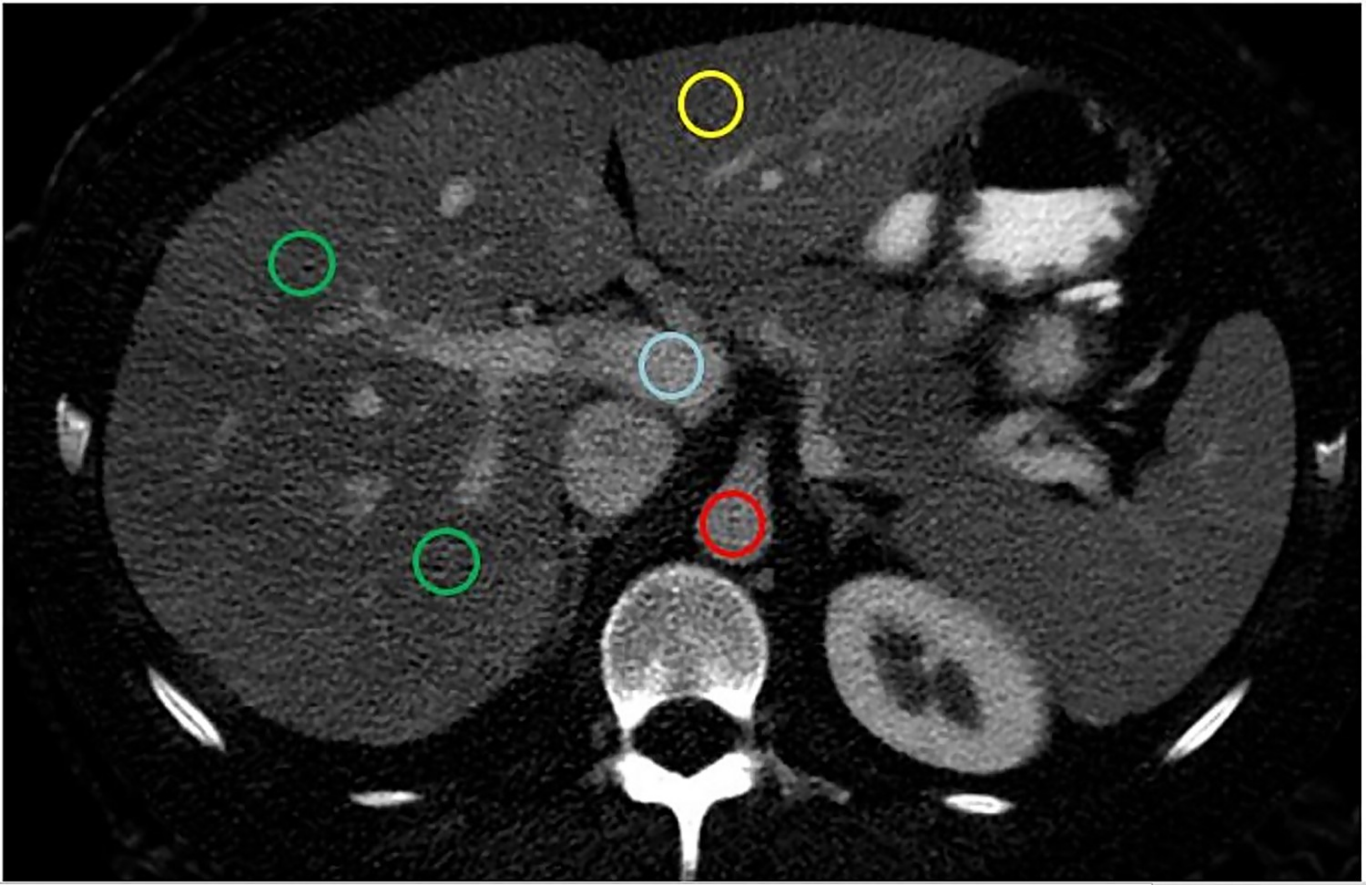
Dual energy CT iodine image, indicating the placement of the ROIs. The 5 mm thick slice of a DECT iodine image in portal venous phase image at the level of the coeliac axis shows five ROIs, all with an approximate area of 1.5 cm^2^. Two ROIs (green) are placed in the right hepatic lobe in segments 4b and 7, one ROI (yellow) in the left hepatic lobe in segment 3, one ROI (blue) in the portal vein, and one in the aorta (red).
